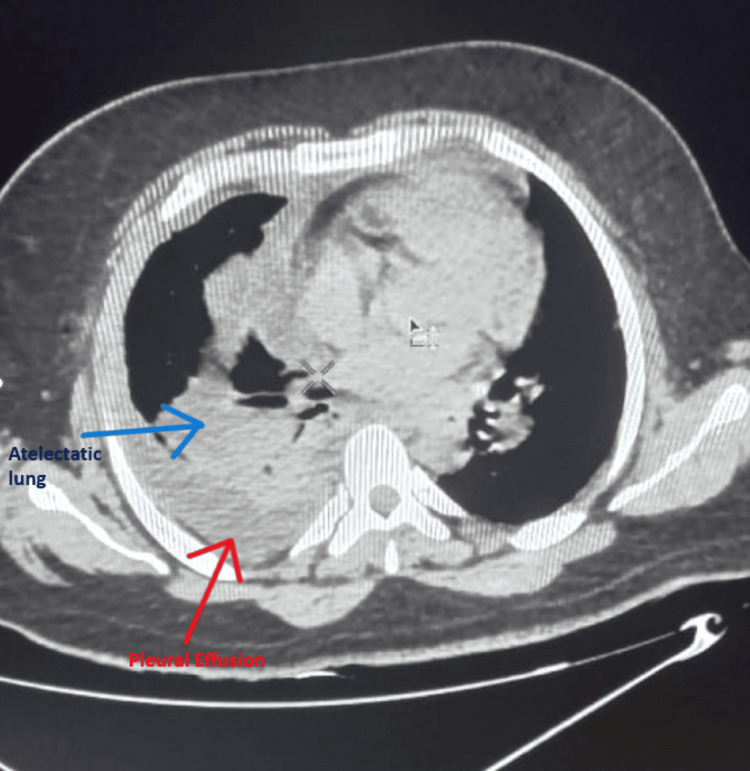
Axial CT chest demonstrating right-sided pleural effusion with associated pulmonary atelectasis Axial noncontrast CT chest demonstrating a right-sided pleural effusion (red arrow) with associated atelectasis of the underlying right lung parenchyma (blue arrow). The effusion occupies the right pleural space with passive collapse of the adjacent lung CT: computed tomography
